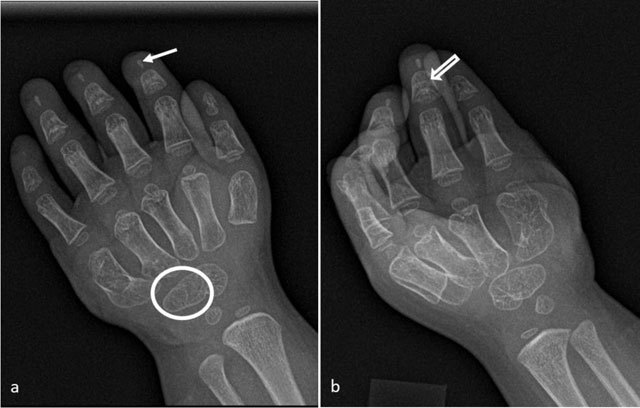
Radiographs of the left hand: A. Posteroanterior view showing hypoplasia of the distal phalanges (arrow at the second finger) and fusion of the hamate and capitate bones (circle). B. Oblique view showing cone-shaped epiphysis of the middle phalanges (open arrow at the fourth finger).
